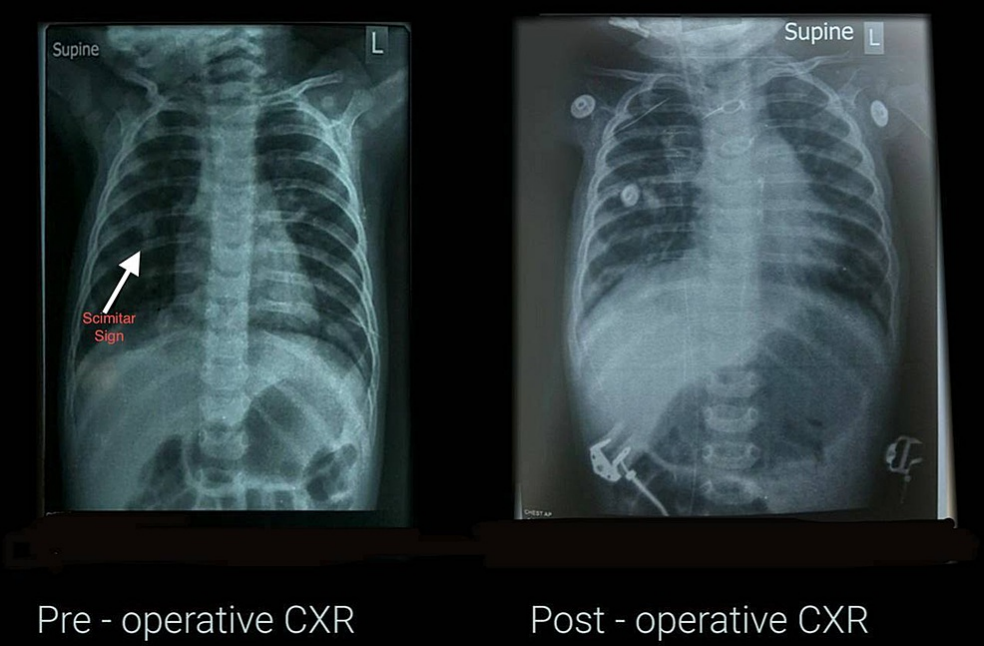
Preoperative (scimitar sign) and postoperative chest radiograph
